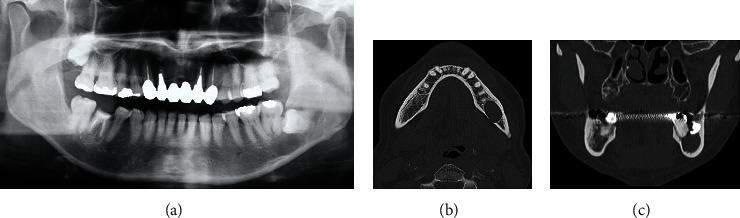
Photoradiograph of the impaction of the left mandibular wisdom tooth and surrounding cystic radiolucent image: (a) orthopantomogram; (b) computed tomography of axial image; (c) computed tomography of sagittal image.
